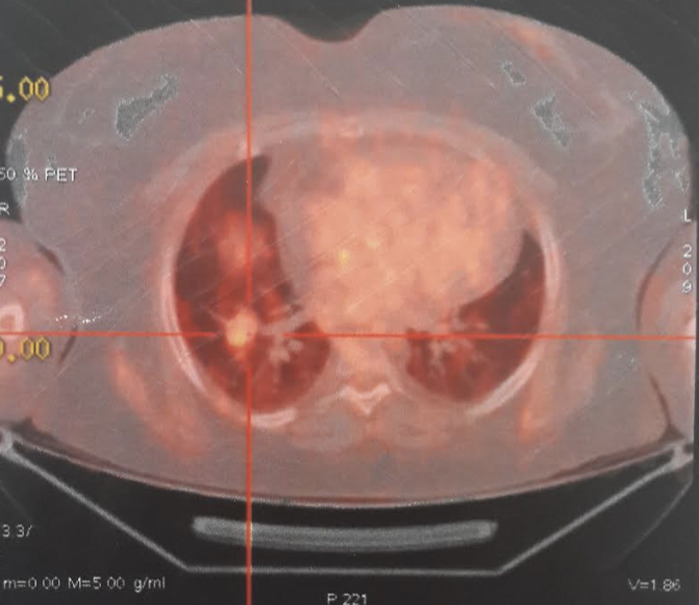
foyer pulmonaire nodulaire actif pathologique sur le 18 FDG PET, de localisation lobaire inférieure droite
